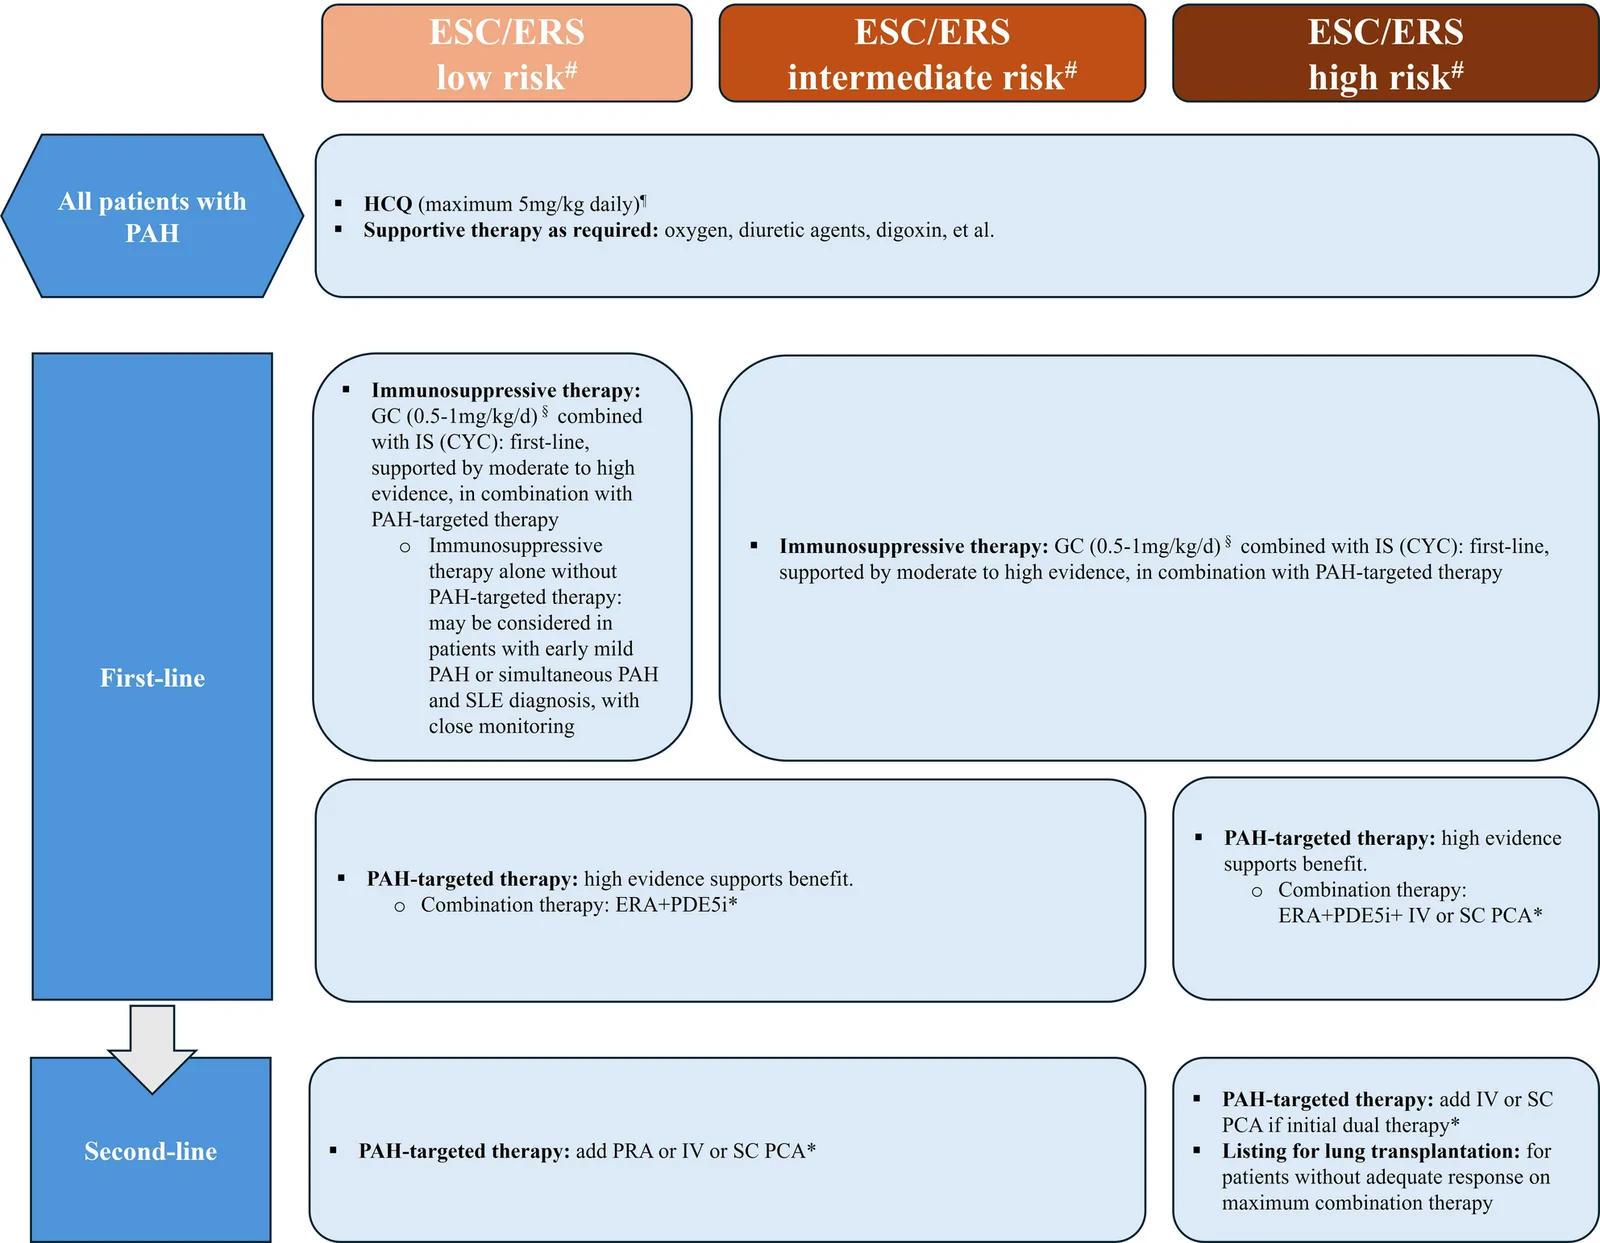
Treatment algorithms for PAH in SLE patients. # The risk stratification is based on a multiparametric approach according to the 2022 ESC/ERS PAH recommendations [105] and the PAH treatment algorithm from the 7th World Symposium on PH [106]. The comprehensive model includes clinical symptoms, WHO-FC, 6MWD, echocardiography, cardiac MRI, and hemodynamics, and the simplified model includes WHO-FC, 6MWD, and BNP/NT-proBNP [105]. * Combination strategy refers to the literature review, the 2022 ESC/ERS Guidelines for the diagnosis and treatment of PH [105], and the PAH treatment algorithm from the 7th World Symposium on PH [106]. ¶ HCQ is recommended as background/baseline therapy for all SLE patients unless contraindicated, according to current guidelines. §The historical data often used uniform, higher initial GC doses. However, the current best practice requires stratifying GC dosing by disease severity. Abbreviation: CYC: cyclophosphamide; ESC: European Society of Cardiology; ERA, endothelin receptor antagonist; ERS: European Respiratory Society; GC: glucocorticoids; HCQ: hydroxychloroquine; IV: intravenous; PAH: pulmonary arterial hypertension; PCA: prostacyclin analogs; PDE5i, phosphodiesterase 5 inhibitor; PH: pulmonary hypertension; PO: per os; PRA, prostacyclin receptor agonist; PVR: pulmonary vascular resistance; SC: subcutaneous; sGCs, soluble guanylate cyclase stimulator; SLE: systemic lupus erythematosus
